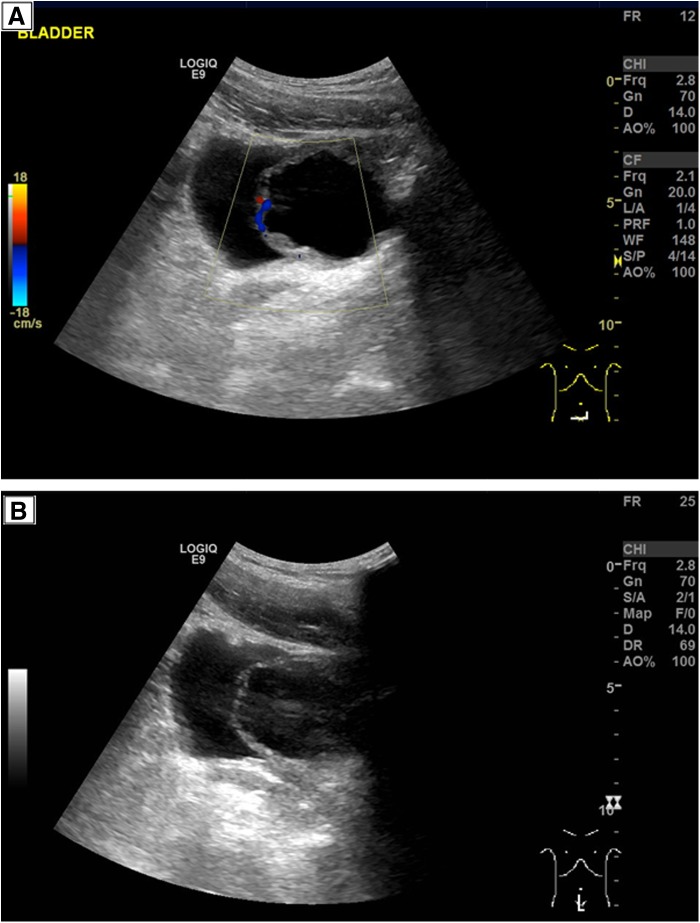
(A, B) Abdominal ultrasound scan showing the cystic lesion and thickened bladder wall.
